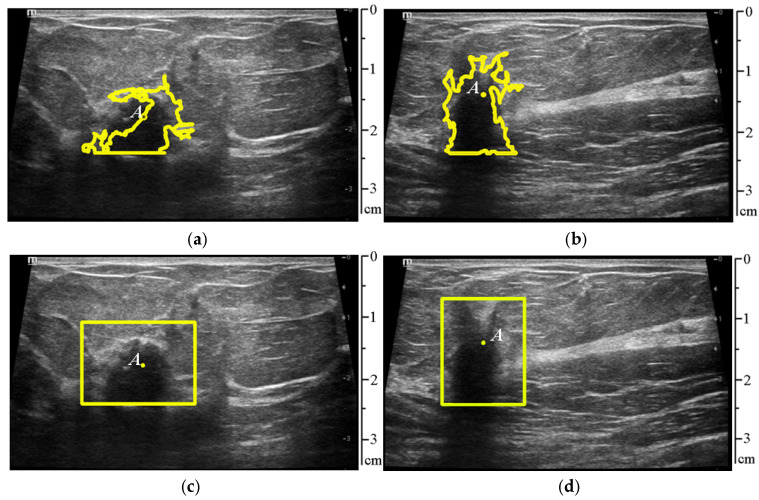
The result of identifying unclassified objects in the frames of ultrasound video sequences corresponding to Figure 1. (a,b) Show the highlighted objects after additional shape filtering, (c,d) show the rectangular areas circled around them, which will be the ROI.
